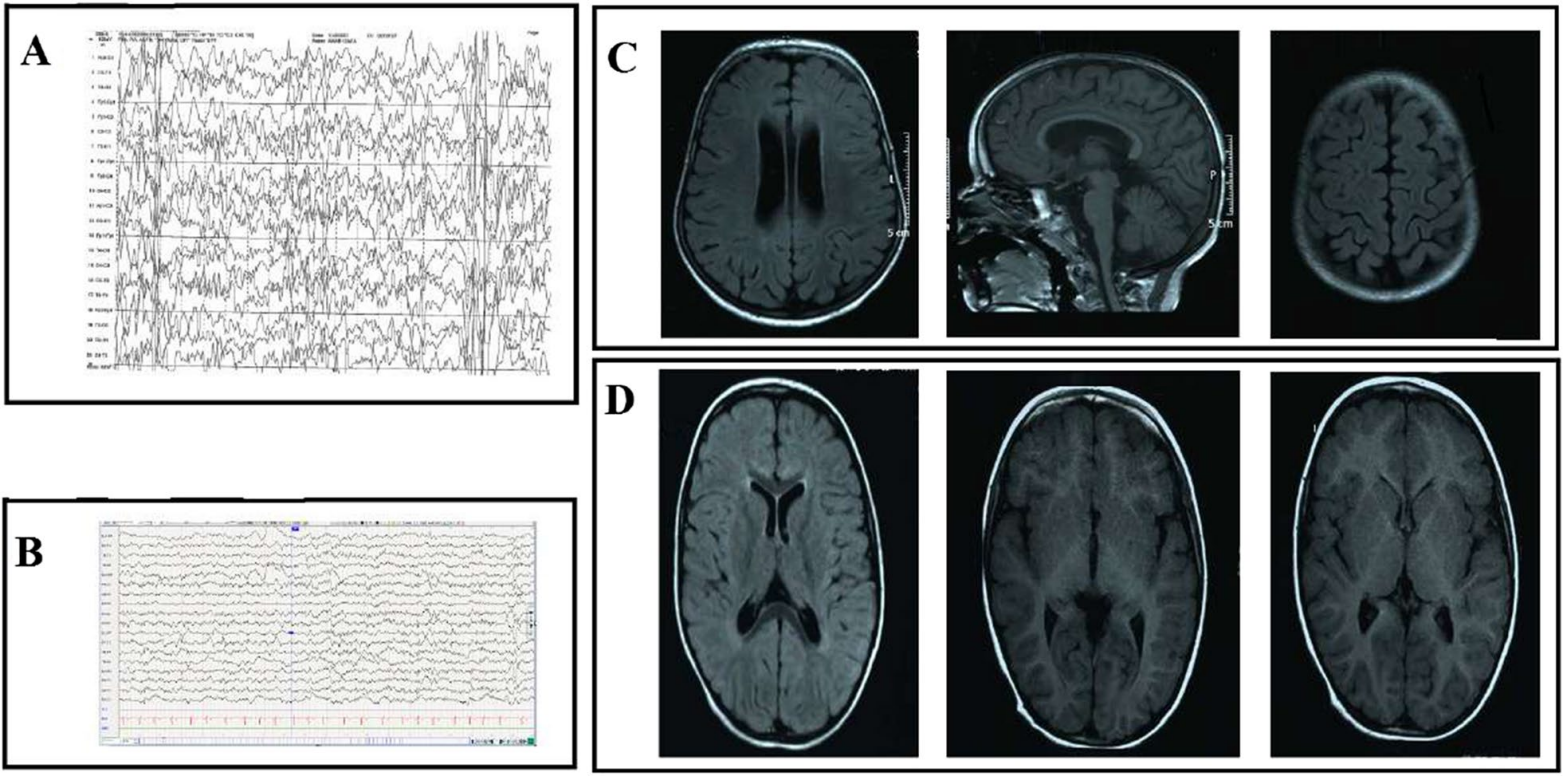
a Sleep (EEG) showing an aspect of hypsarrhythmia: high amplitude and irregular waves and spikes in a background of chaotic and disorganized activity. b Awake EEG showing multifocal spikes discharges. c, d Coronal section of brain MRI from patients carrying the p.Gly471Arg mGlu7 variant. c Coronal section of brain MRI of VI4 patient at the age of 10 years showed a cortical and sub-cortical atrophy. d Coronal section of brain MRI of VI1 patient at the age of 2 years showed a discrete hyper T2 of posterior SB
